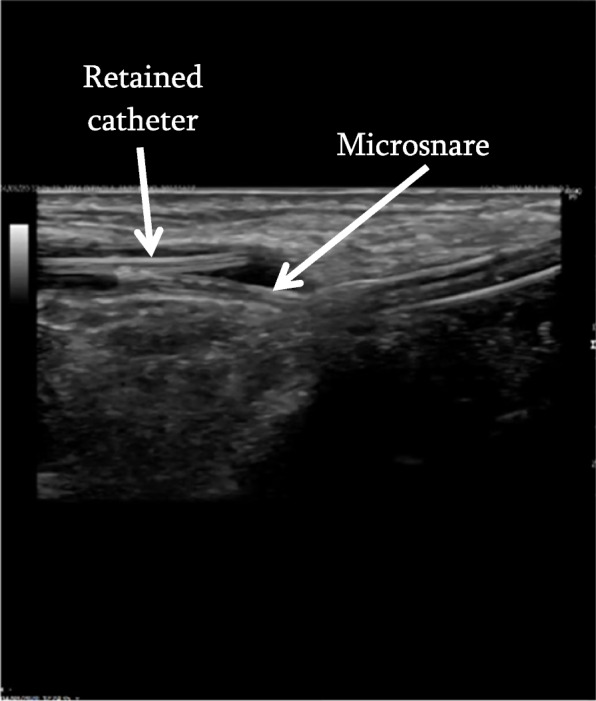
Ultrasound image demonstrating microsnare capturing the retained arterial line fragment
